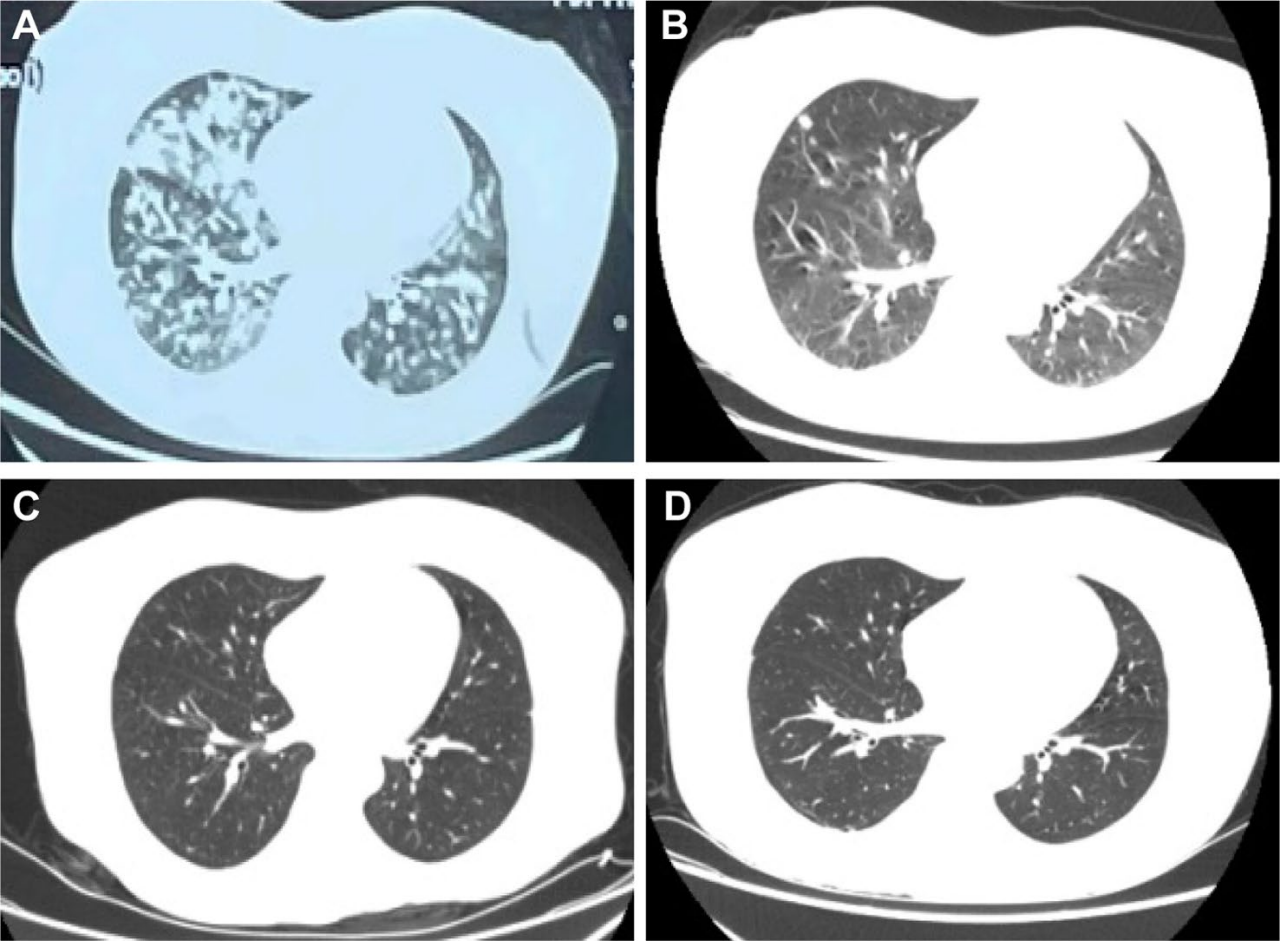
Chest CT at different stages. (A) In early 2023, a local lung CT scan showed multiple nodular increased density shadows in both lungs. (B) Five weeks later (1 week of antifungal treatment), a lung CT scan showed that the bilateral lung lesions were significantly less and smaller than before. (C) Nine weeks after the incident (1 month of antifungal treatment), lung CT showed that the abscess lesions were smaller than last time. (D) More than 8 months after the incident (more than 7 months of antifungal treatment), the bilateral lung lesions still exist
